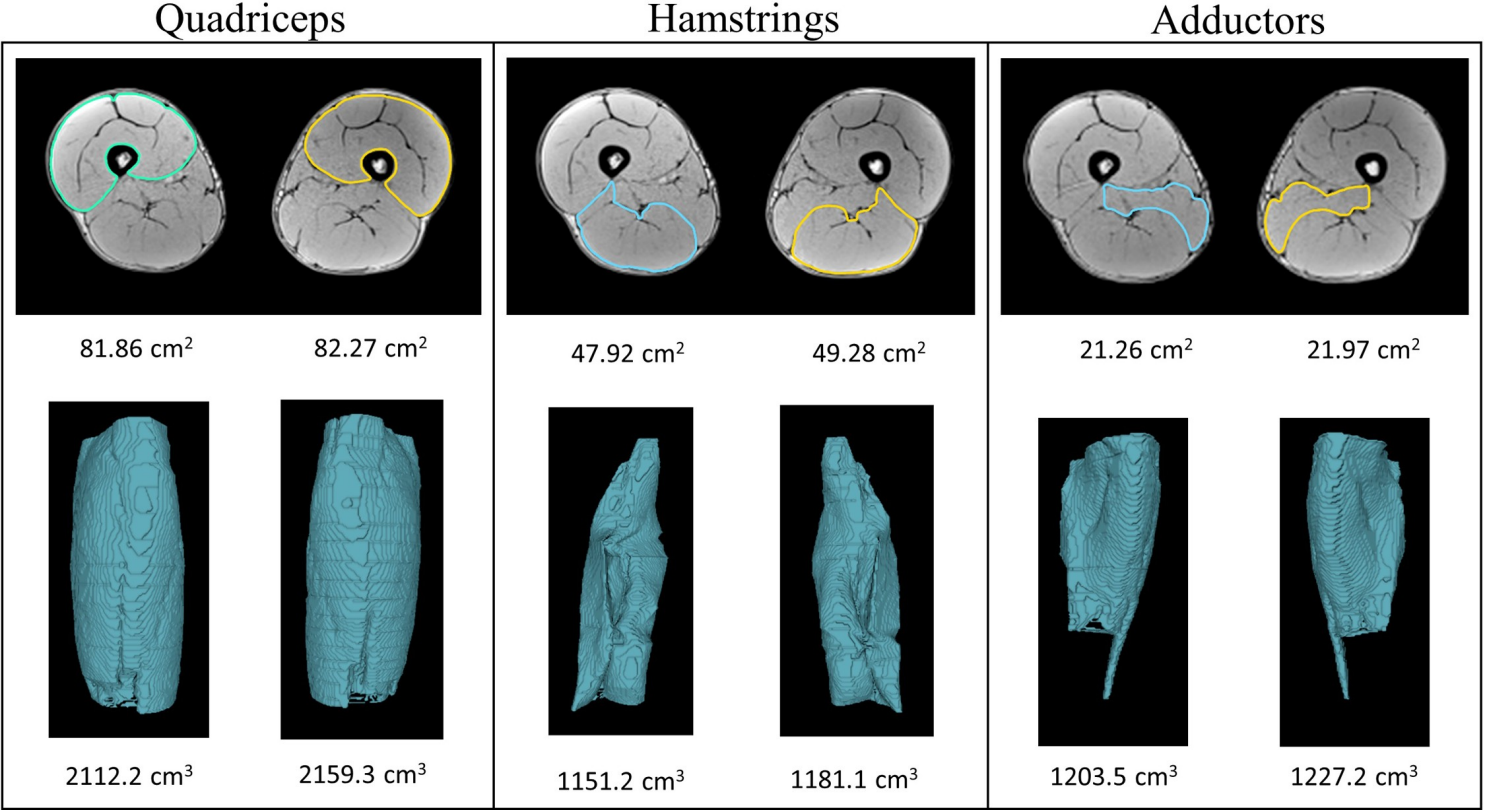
Quadriceps, hamstring and adductor muscle volume assessment. Representative magnetic resonance imaging scans, mid-thigh, of subject no. 10 (male) used for measuring cross-sectional area of quadriceps, hamstrings and adductors, and their respective muscle volume reconstructions.
